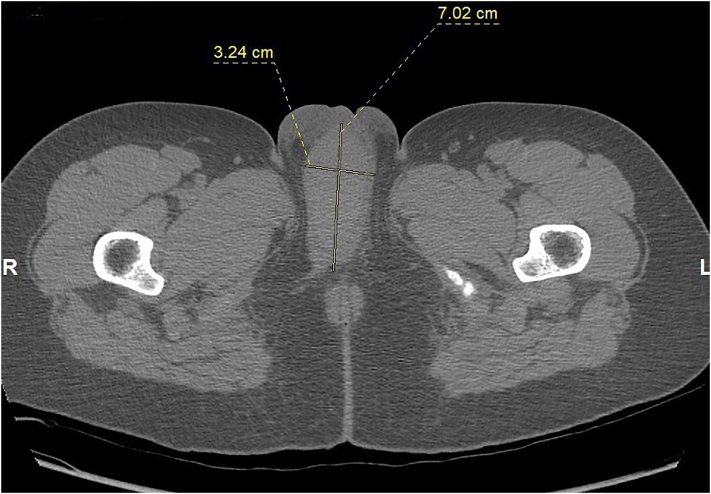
The axial computed tomography scan shows a large hematoma in the right labium majus with a diameter of 7.02 × 3.24 cm.
